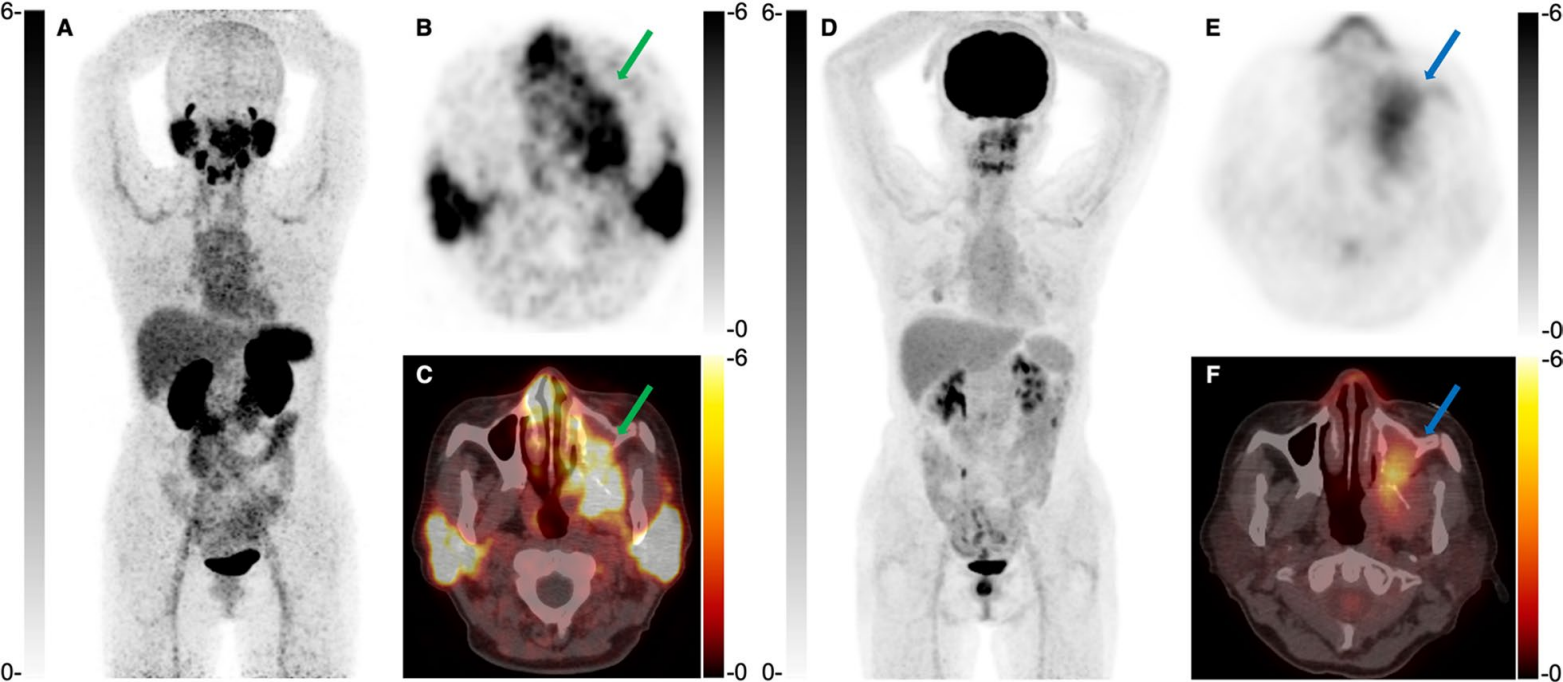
A 53-year-old female patient with primary ACC. Anterior maximum intensity projection (MIP) and axial ^68^ Ga-PSMA PET/CT (A–C) showed increased tracer uptake of the tumor in the left maxillary sinus (green arrow, SUVmax 9.8). ^18^F-FDG PET/CT (D–F) showed a lower uptake (blue arrow, SUVmax 6.3)
